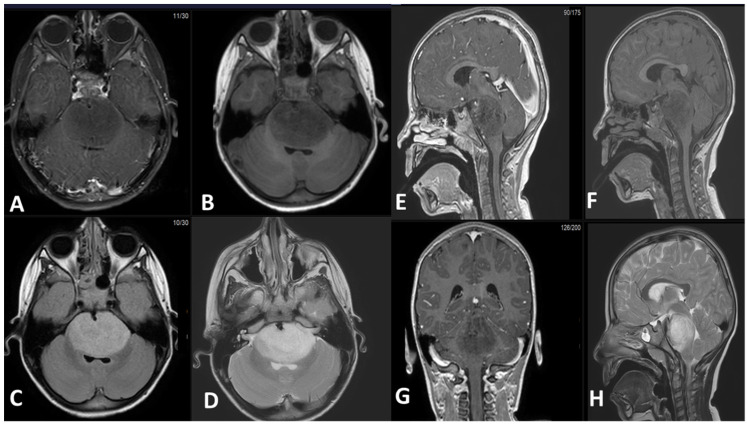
(A) Axial T1-weighted post IV contrast fat saturated sequence. (B) Axial T1-weighted pre-IV contrast sequence. (C) Axial FLAIR sequence post IV contrast. (D) Axial T2 TSE sequence. (E) Sagittal T1-weighted post IV contrast sequence. (F) Sagittal T1-weighted pre-IV contrast sequence. (G) Coronal T1-weighted post IV contrast sequence. (H) Sagittal T2 TSE sequence. FLAIR (fluid attenuation inversion recovery). TSE (turbo spin echo).
